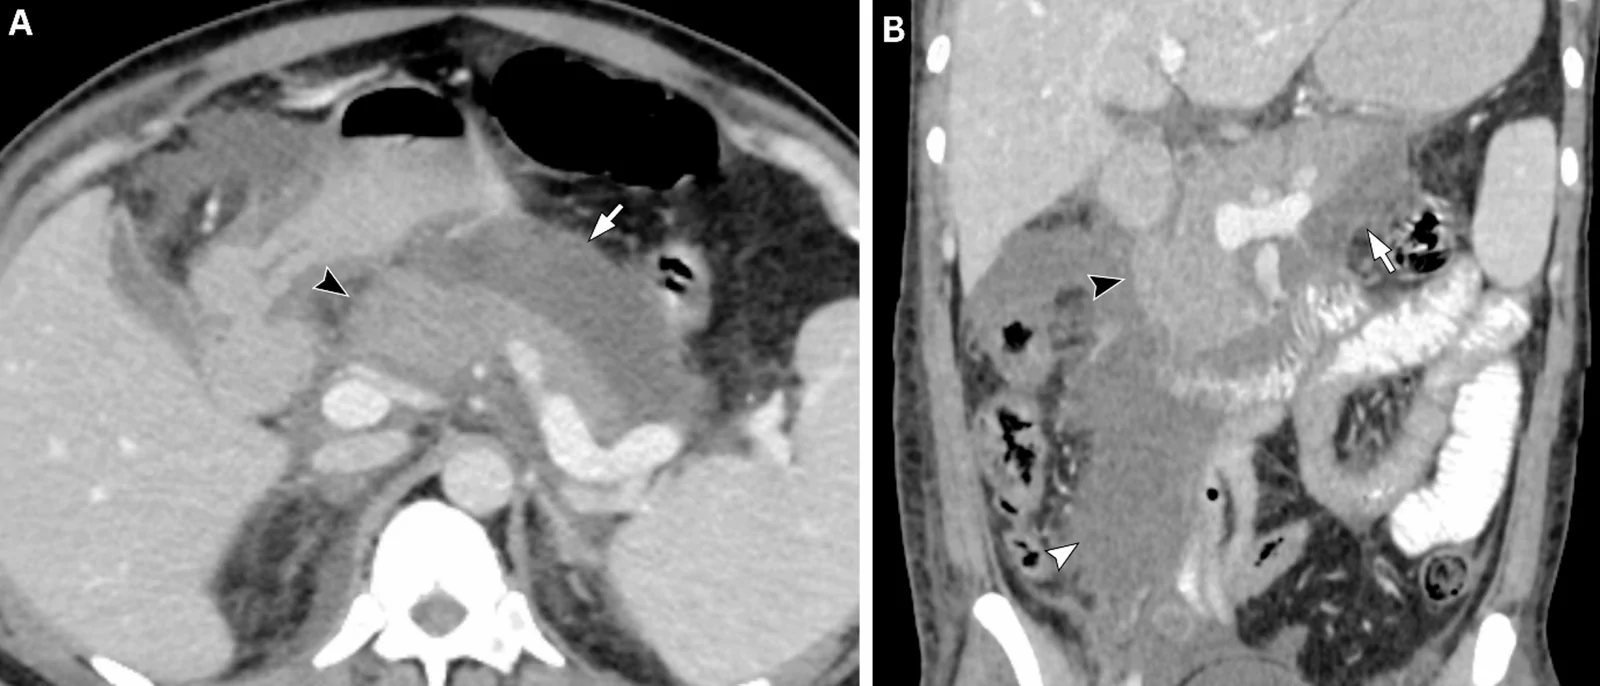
Acute interstitial edematous pancreatitis associated with scrub typhus in a 40-year-old male with serum lipase measuring 1566 U/L. A–B Axial and coronal contrast-enhanced CT (CECT) images show a mildly enlarged, edematous pancreas (black arrowhead), accompanied by acute peripancreatic fluid collection (white solid arrow) and small volume ascites (white arrowhead)
